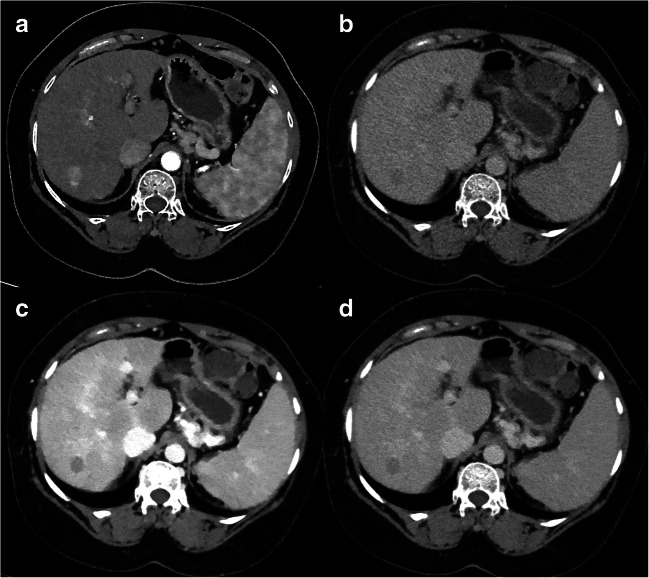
Axial CT images showing an arterially hyper-enhancing lesion in liver segment VII (a) with washout in delayed phase on CI (b), 40 keV (c) and 60 keV (d). However, washout and lesion-to-liver ratio were higher in 40-keV compared to 60-keV images and CIs, resulting in a better visibility
